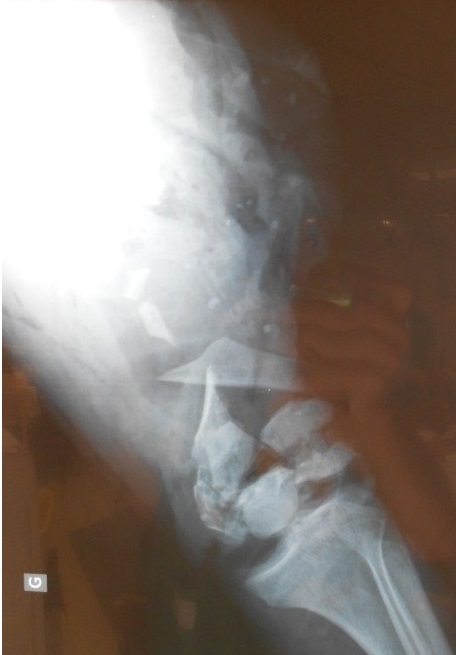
Anteroposterior radiograph of the left knee showing comminuted distal femur fracture with bone loss
